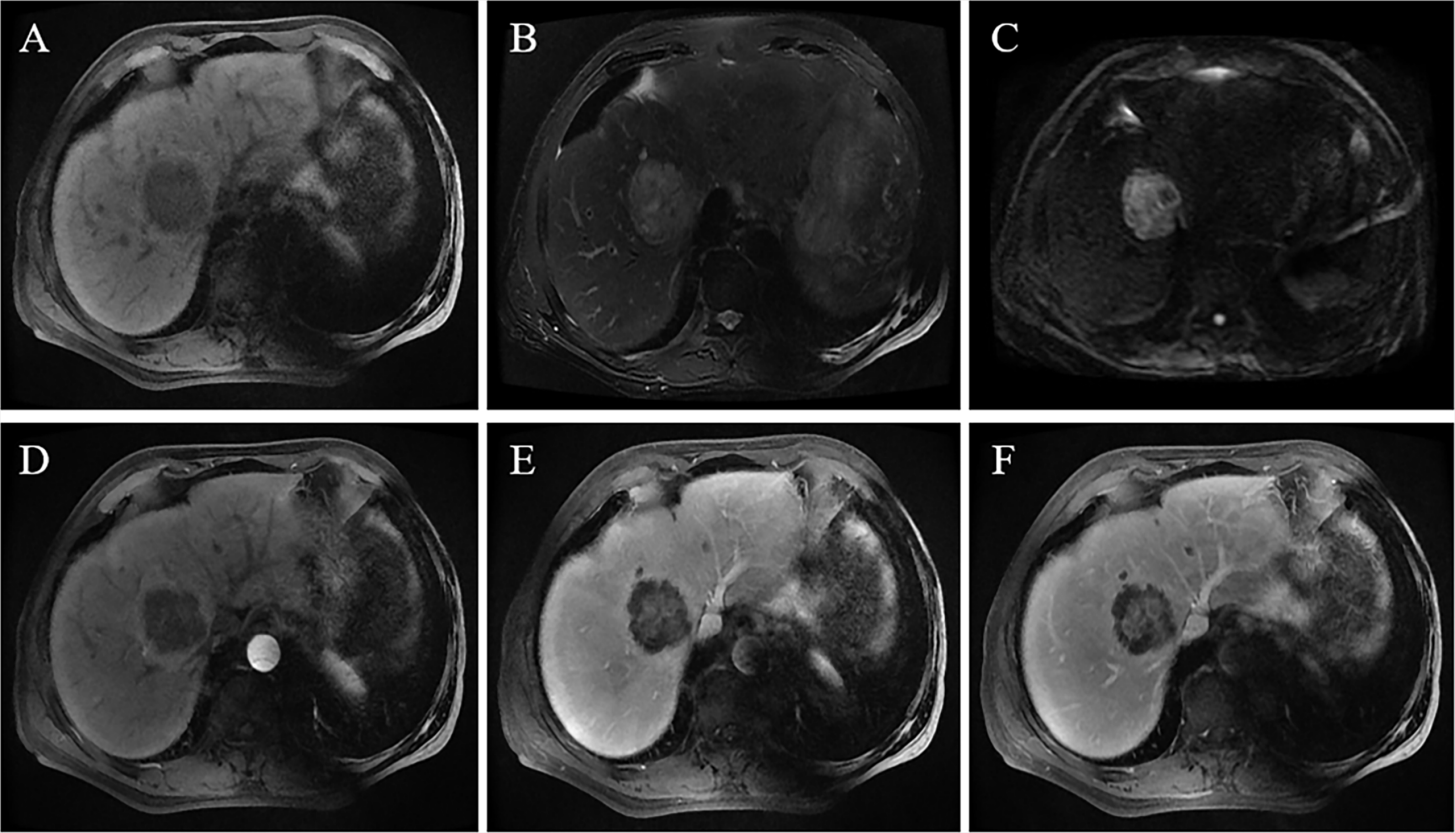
A 69-year-old male patient was accidentally found to have a liver tumor on an annual screening. An MRI scan revealed that the tumor was located in the right lobe and was 5.8 cm in size. The lesion showed a hypointense signal on T1WI (A), slightly hyperintense signal on T2WI, and hepatic capsular retraction (B); the tumor showed a high signal on the DWI sequence with b = 1000 s/mm² (C). On contrast-enhanced MRI, the lesion shows peripheral enhancement in the arterial phase, blurred patchy hyperintense signal in the central region of the tumor (D), and massive enhancement in the central region of the tumor in the portal phase and delayed phase (E, F).
